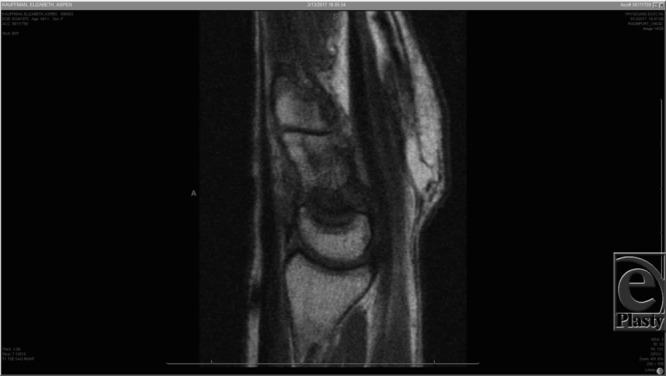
Sagittal MRI slice of the right wrist. The proximal pole of the capitate has a low-intensity signal (Milliez type I).
